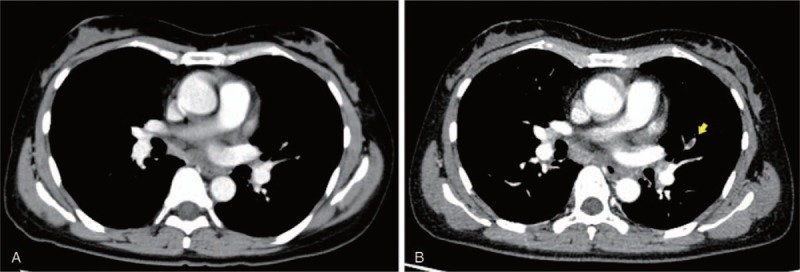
Chest-enhanced CT images; (A) Before pembrolizumab administration (B) On day 7 after administration. Yellow arrow indicates enhancement defect suggesting thrombus formation in the left pulmonary artery. CT = computed tomography.
